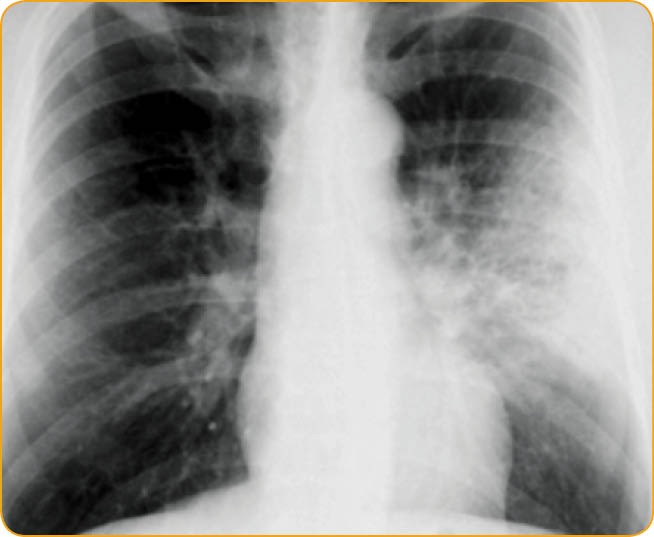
Chest x-ray shows pneumonia.
